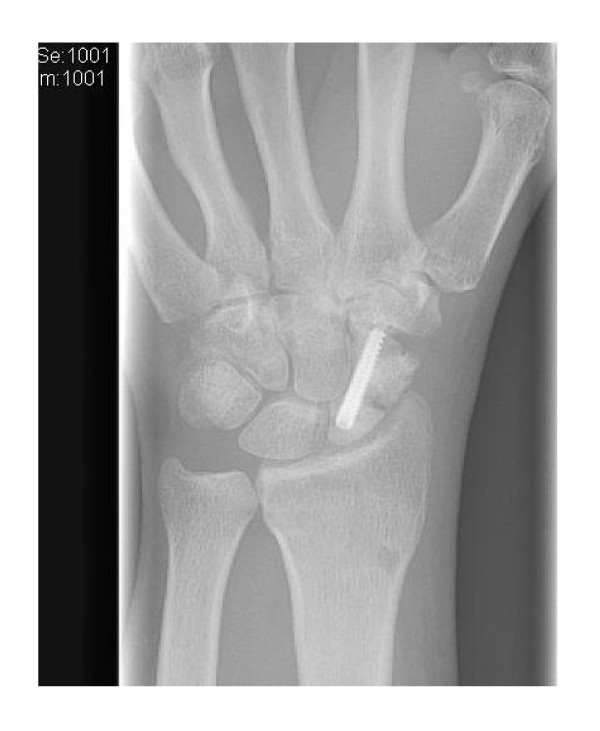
X-ray after percutaneous screw fixation of the scaphoid (patient F).
